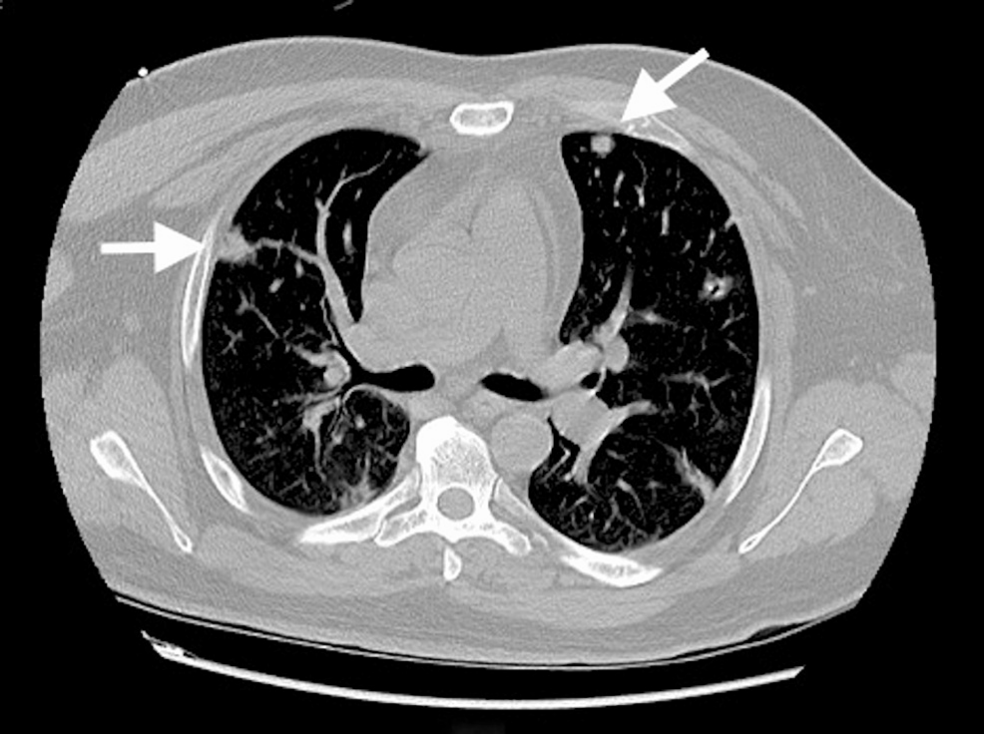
CT chest showing multiple bilateral pulmonary nodules highly suspicious for metastatic disease, measuring up to 1.5 cm in the right upper lobe.
